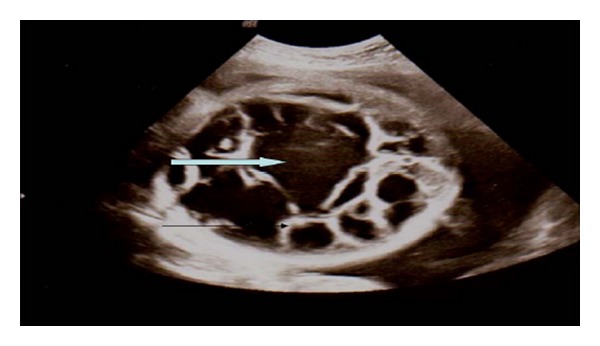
Antenatal ultrasound scan view showing the foetal abdomen and revealed the presence of a large cystic lesion with multiple thick septation (black arrow) containing fluid and fine echoes (block arrow).
